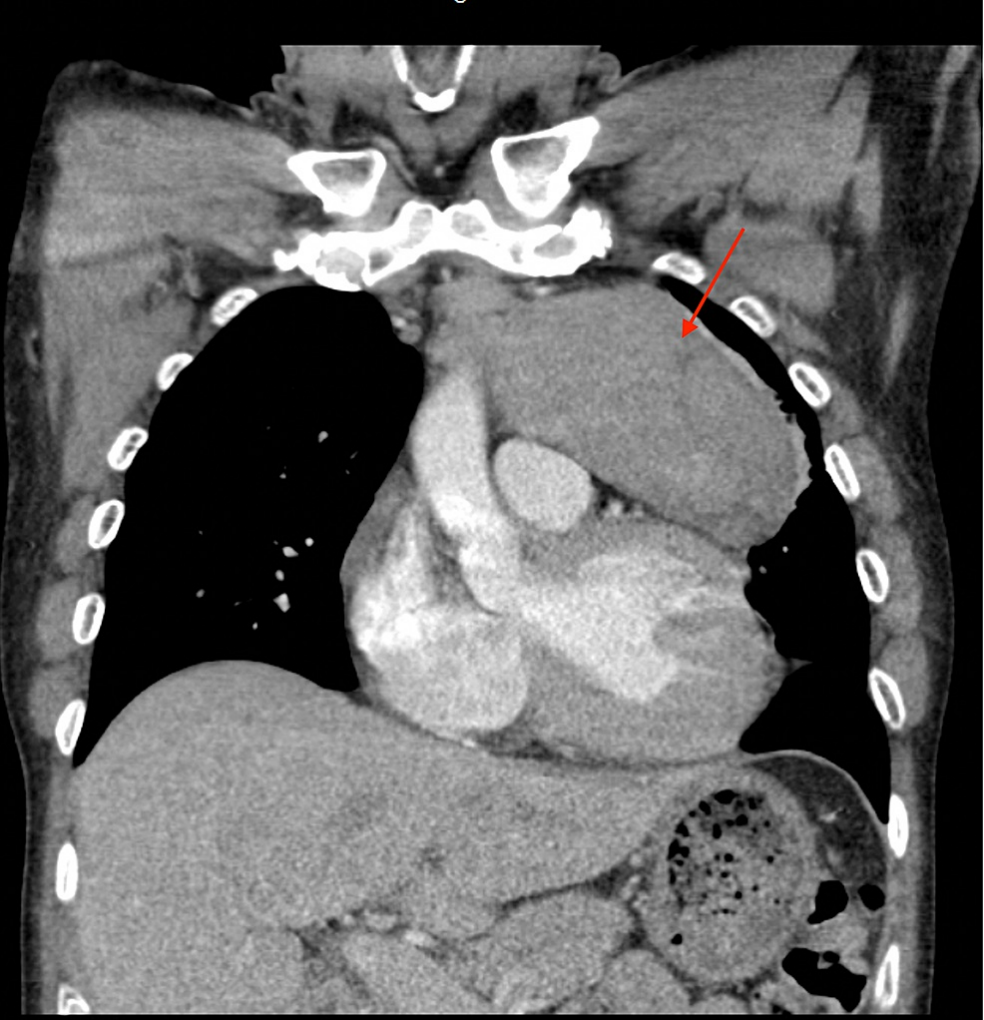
Chest-CT with contrast, coronal view, 10.3 cm anterior mediastinal mass with two adjacent enlarged mediastinal lymph nodes
